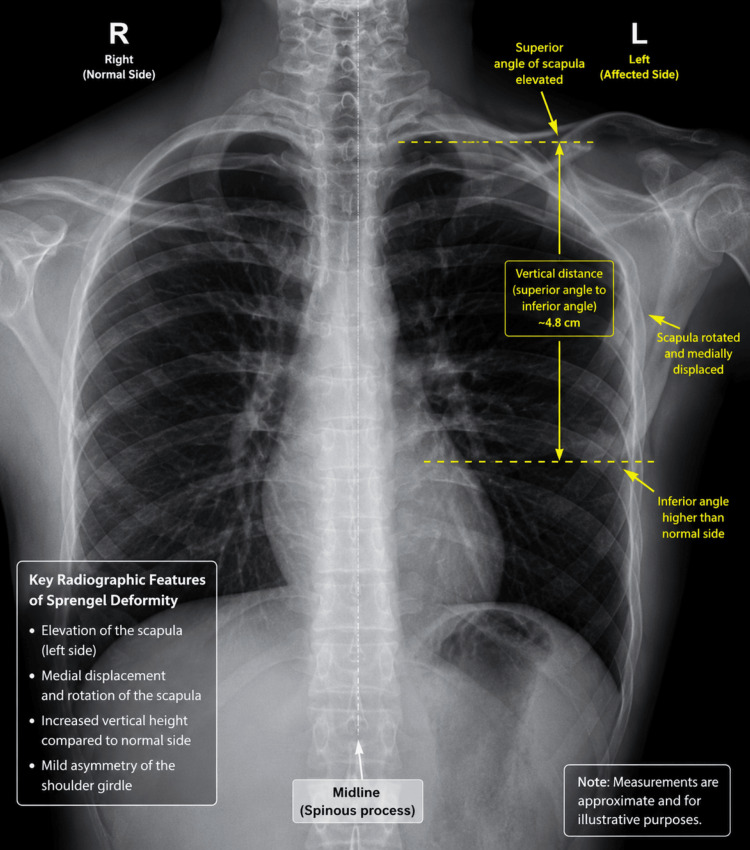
Chest radiograph showing elevated left scapula consistent with Sprengel's deformity and mild asymmetry of the shoulder girdle
